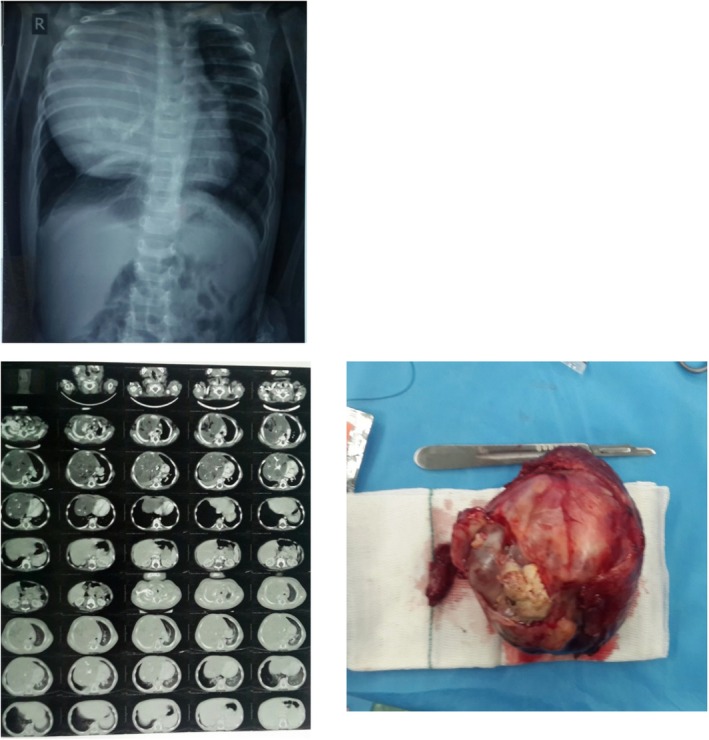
Chest X‐ray (left), lung CT scan (middle) of a huge intrathoracic mass, which was extracted by our method of surgery. The right picture shows the completely resected mass, whose pathological findings were compatible with the diagnosis of teratoma.
